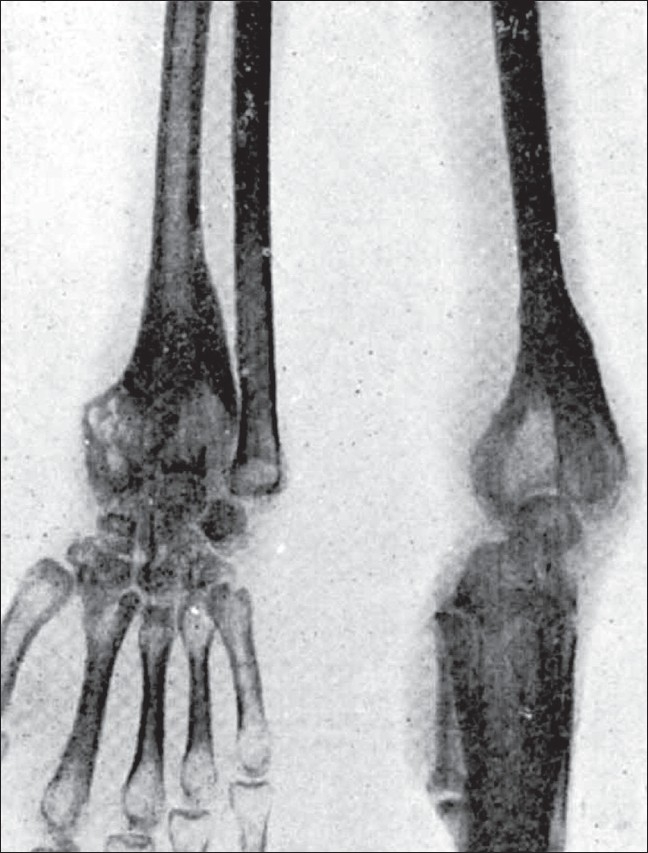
Radiograph of an osteosarcoma. There is expansion; but the abundant periosteal reaction and invasion of shaft with the loss of curved line of demarcation distinguish it from osteoclastomaEccentric situation in the bone.Curved line of graded density definitely, demarcating the tumour from the normal bone.Absence of periosteal reaction.Extension into adjacent tissue and bones.The relative resistance of the sub-articular bone.
